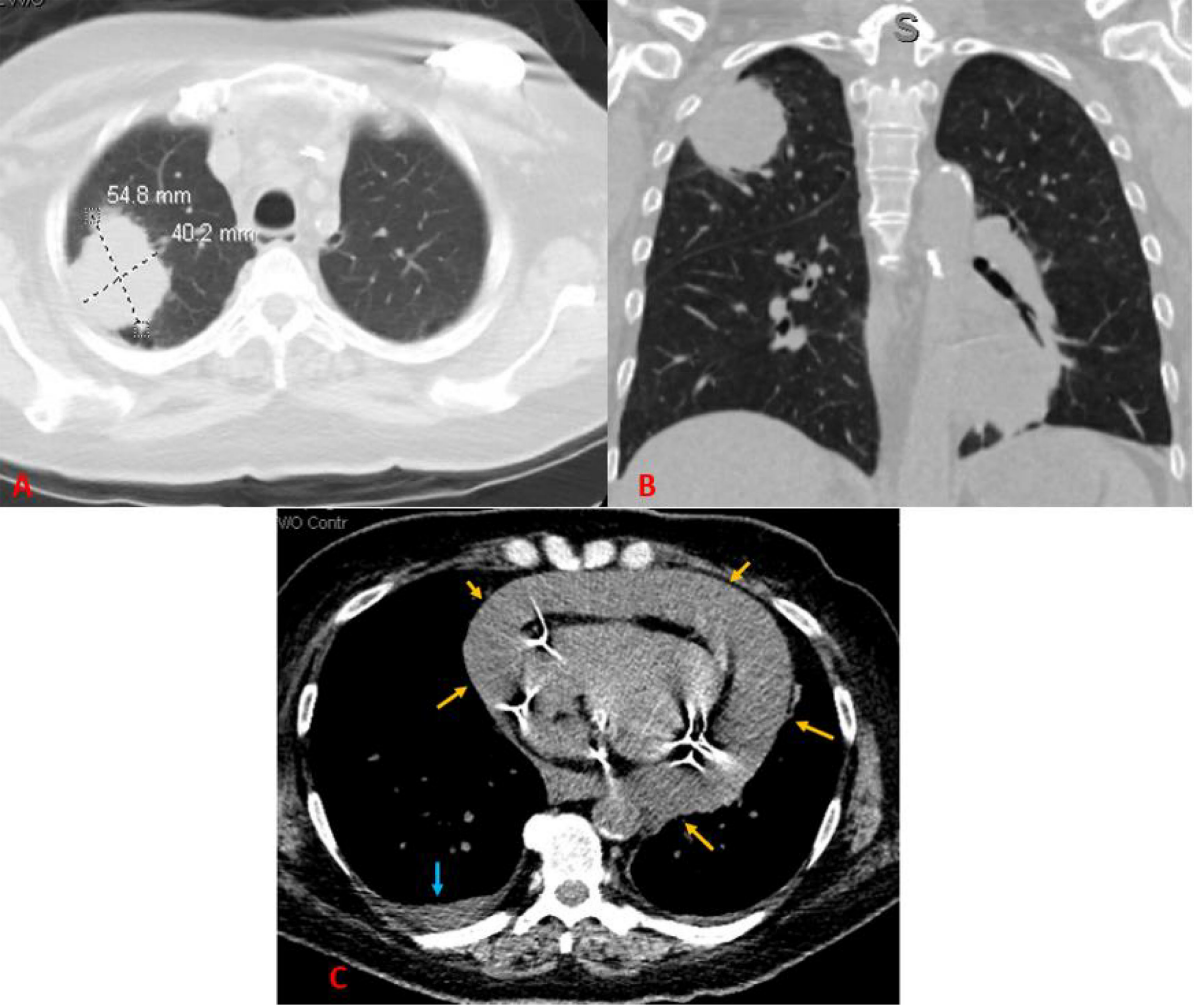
A CT scan of the chest without contrast. (A) Axial image showing a right upper lobe mass measuring approximately 5.5 × 4.0 cm in maximum axial dimensions. (B) Coronal image showing the right upper lobe mass contacting the pleura. (C) Axial image showing a large pericardial effusion (yellow arrows) and a small right pleural effusion (blue arrow).
